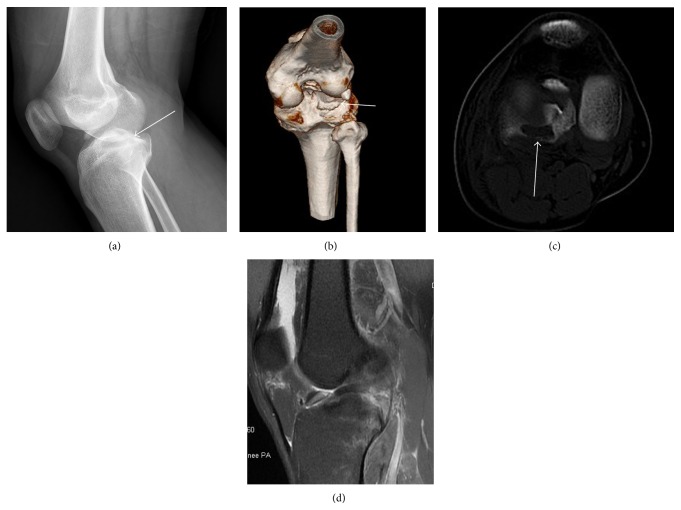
Imaging examinations of the second patient. The X-ray image (a) shows a posterolateral tibial plateau fracture (white arrow). In the CT scans (b and c), a cavity was formed at the lateral location of posterolateral tibial plateau fracture (white arrow). In the MRI image (d), ACL rupture and lateral meniscus tear were confirmed. Other ligaments were not found by the MRI scan.
